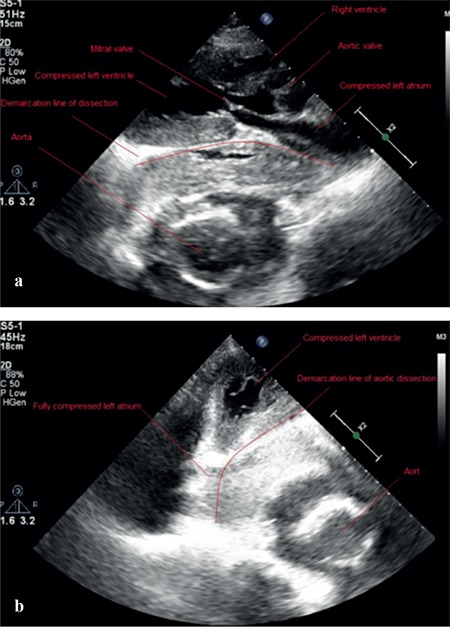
a-b. Long axis view of the transthoracic echocardiographic image showing the dissecting aortic aneurysm causing the compression of the left heart chambers when the patient was evaluated for the first time (a), Four-chamber view of the transthoracic echocardiographic image showing the dissecting aortic aneurysm causing the compression of the left heart chambers before exitus (b).
